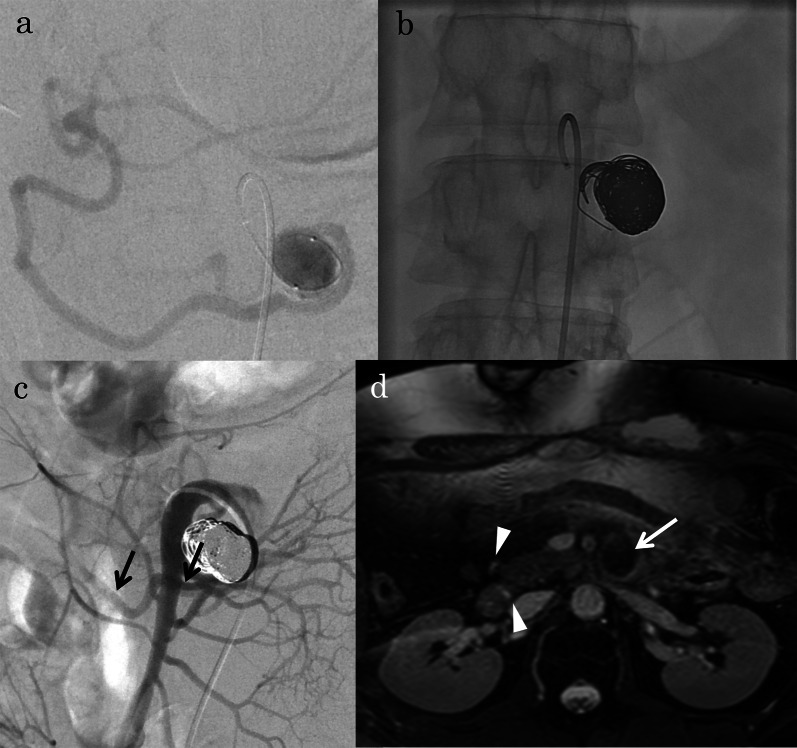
Images from Case 2. a Intra-aneurysmal angiogram immediately before coil embolization showing opacification of the distal inferior pancreaticoduodenal artery (PDA). b Fluoroscopic images during coil embolization showing coil packing of the inferior PDA aneurysm (PDAA). c Post-embolization angiogram via the superior mesenteric artery showing complete occlusion of the aneurysm with excellent preservation of the inferior PDA (black arrows). d Non-contrast-enhanced magnetic resonance imaging after treatment showing disappearance of the flow in the PDAA (white arrow) and decreased thickness of the pancreatic arcades (white arrowheads)
